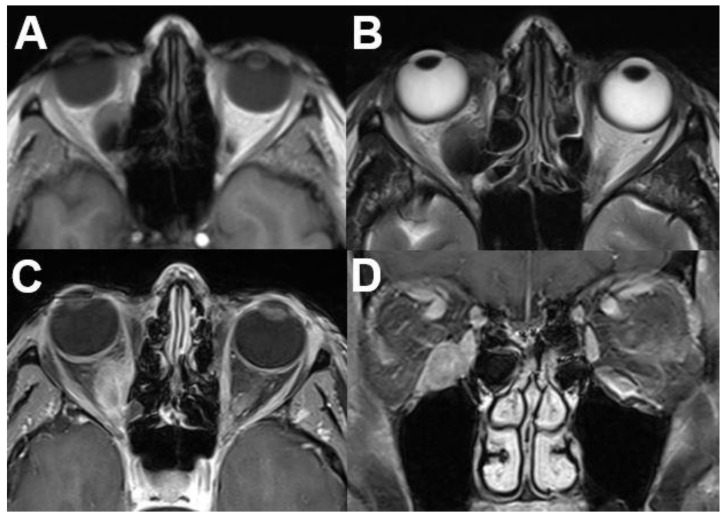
Metastasis.* MRI of a 56-year-old female patient with breast cancer. MRI shows an ill-defined tumor in the right-sided medial orbit showing T1-hypointense (A) and T2-hypointense (B) signal and inhomogeneous contrast enhancement (C,D). The tumor is in contact with the optic nerve and infiltrates the medial and inferior rectus muscle.
